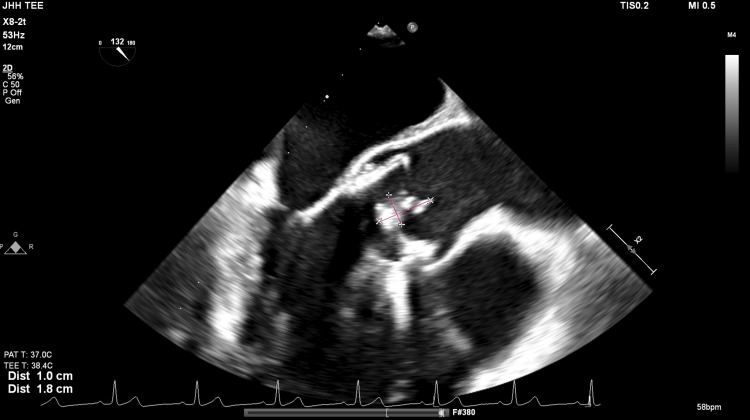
TEE showing 1.8 × 1.0 cm mobile vegetation on the bioprosthetic aortic valve. TEE, transesophageal echocardiography
